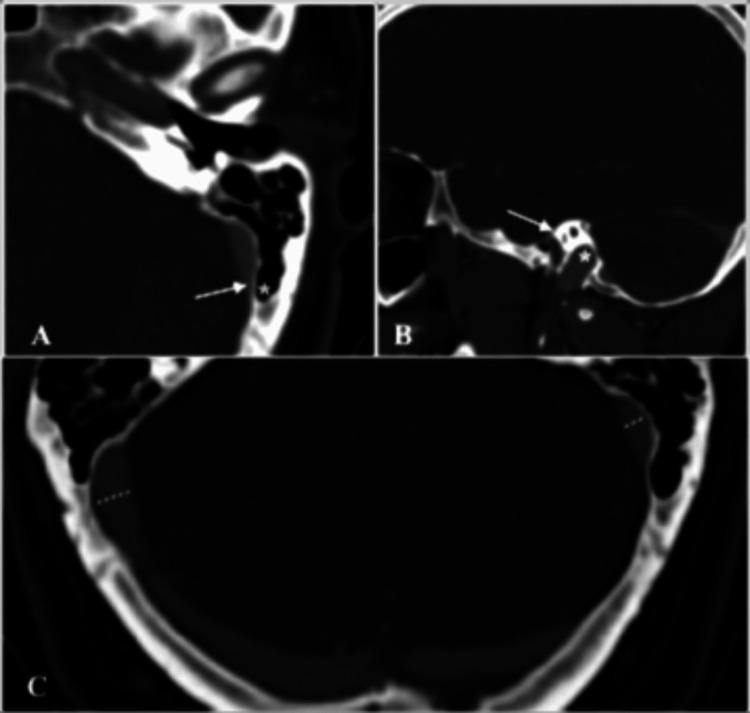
Computed tomography angiographies of the head A: axial view of the left temporal bone demonstrates a type 4 level of pneumatization of the mastoid with an aerated mastoid cell (asterisks) extending beyond the posterior limit of the sigmoid sinus (arrow); B: sagittal view of the right temporal bone demonstrates a type 3 jugular bulb with its dome (asterisks) located above the inferior margin of the posterior SCC (arrow); C: axial view of the posterior fossa demonstrates a right-side dominant SS (dotted lines ratio > 1.5), a type 2 pneumatization on the right and type 4 pneumatization on the left.
